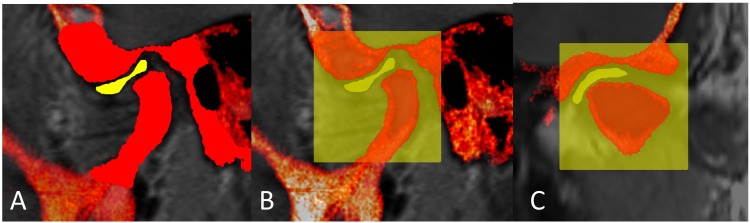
Tissues segmentation in MRI(gray)-CBCT(red) registered images. A: Oblique sagittal PD-weighted MRI-CBCT registered image showing outlined/segmented articular disc (yellow) and condylar head and TMJ structure of the temporal bone (bright red). B: A yellow 3D cropping box of about 2.5cm^3^ in dimensions was manually drawn to export the cropped TMJ structures only as STL files. C: Oblique coronal PD-weighted MRI-CBCT registered image showing the medio-lateral dimensions of the same cropping box highlighting the cropped TMJ structures.
